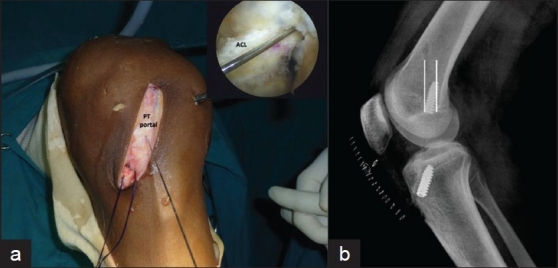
(a) Guide wire through PT portal showing parallel wire in the arthroscopic image (inset) in the left knee. (b) Lateral X-ray of knee showing interference screw near parallel to femoral tunnel (parallel white lines depict outline of femoral tunnel)
